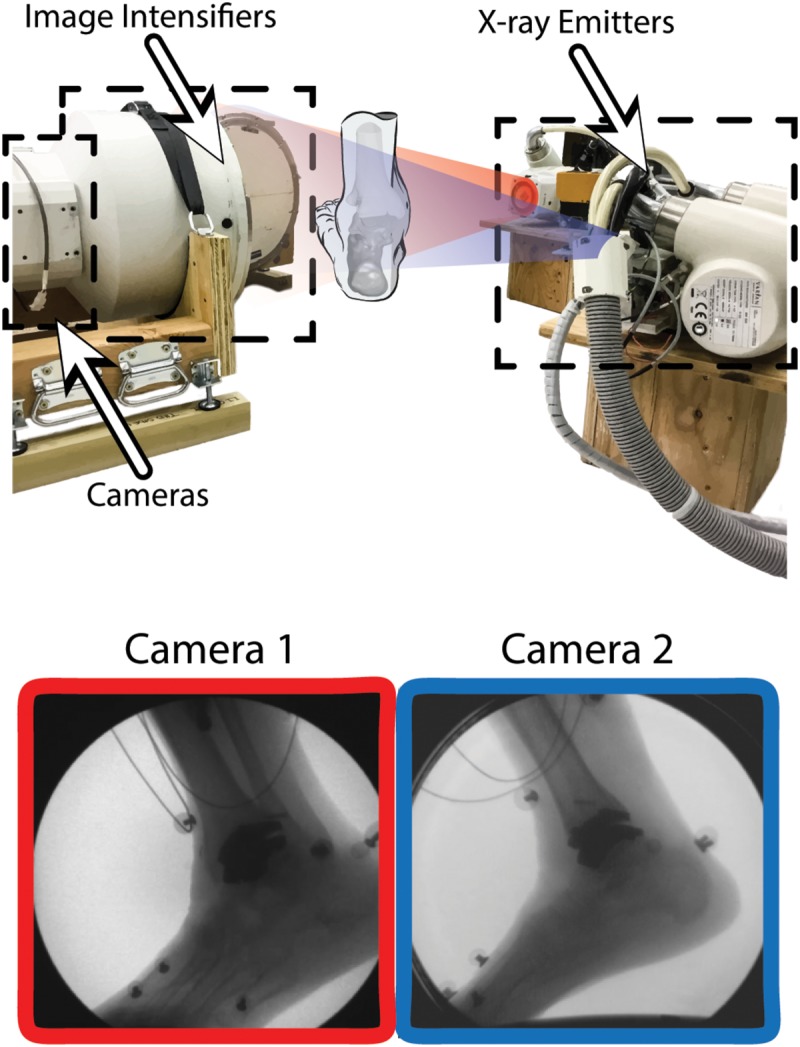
Dual fluoroscopy (DF) system setup for overground walking with two X-ray emitters and two image intensifiers with high-speed cameras oriented ≈90 degrees from one another. Two synchronized DF views (Camera 1 and 2) are shown during overground walking toward the toe-off phase of gait. Wires from electromyography sensors can be seen in the DF image.
